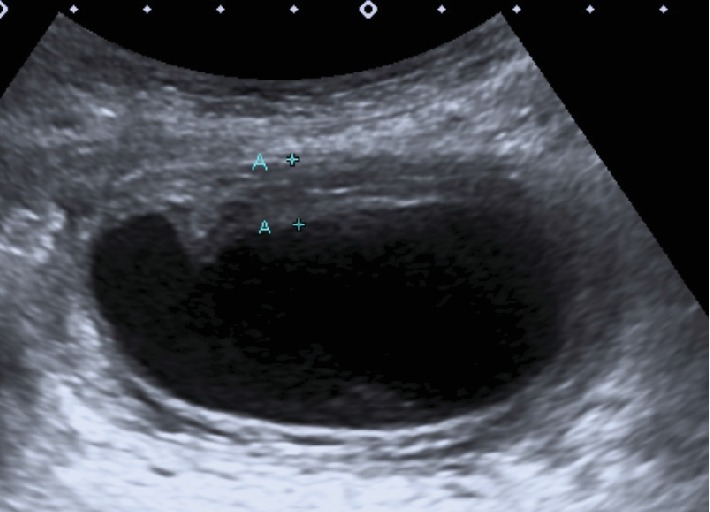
Gray-scale ultrasound depicting lamellated wall thickening (A+) of the gallbladder without cholelithiasis.
